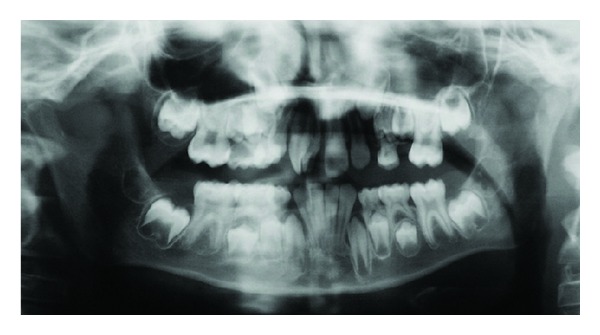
Panoramic radiography. Notice the fused tooth with two root canals.
